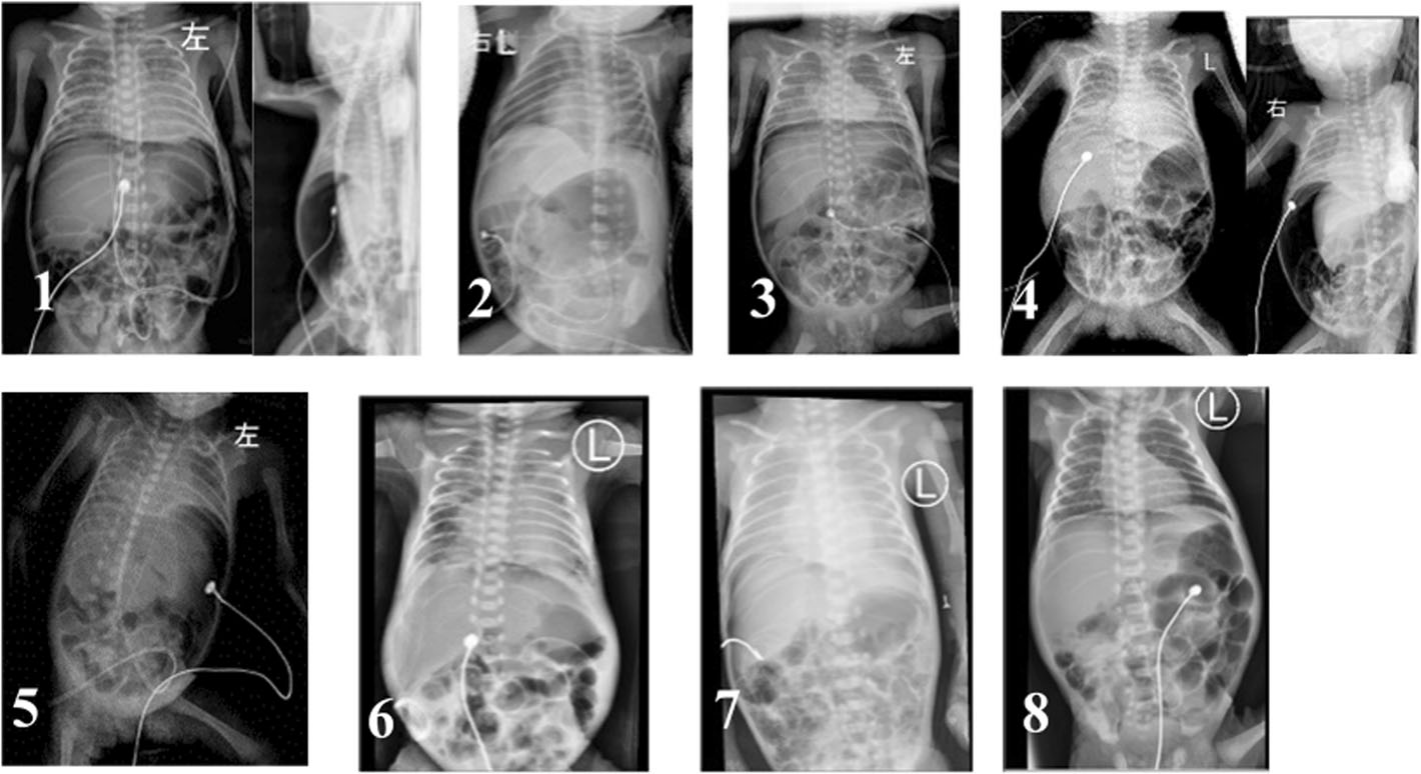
Diagnostic X-rays of pneumoperitoneum in all 8 cases
